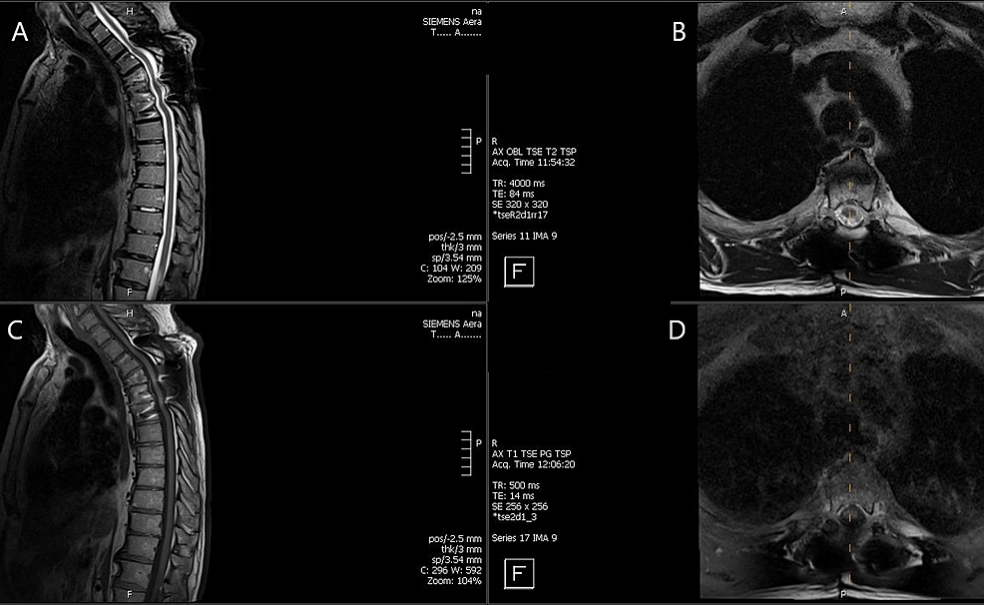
MRI of the thoracic spine 18 months following surgery A and C: Sagittal MRI T2 and T1 without contract revealing complete resolution of the mass lesion with artifact from instrumentation but no mass effect or cord compression B and D: Axial T2 and T1 weighted images with no epidural mass in the T4-T5 region and a resolved prevertebral lesion
